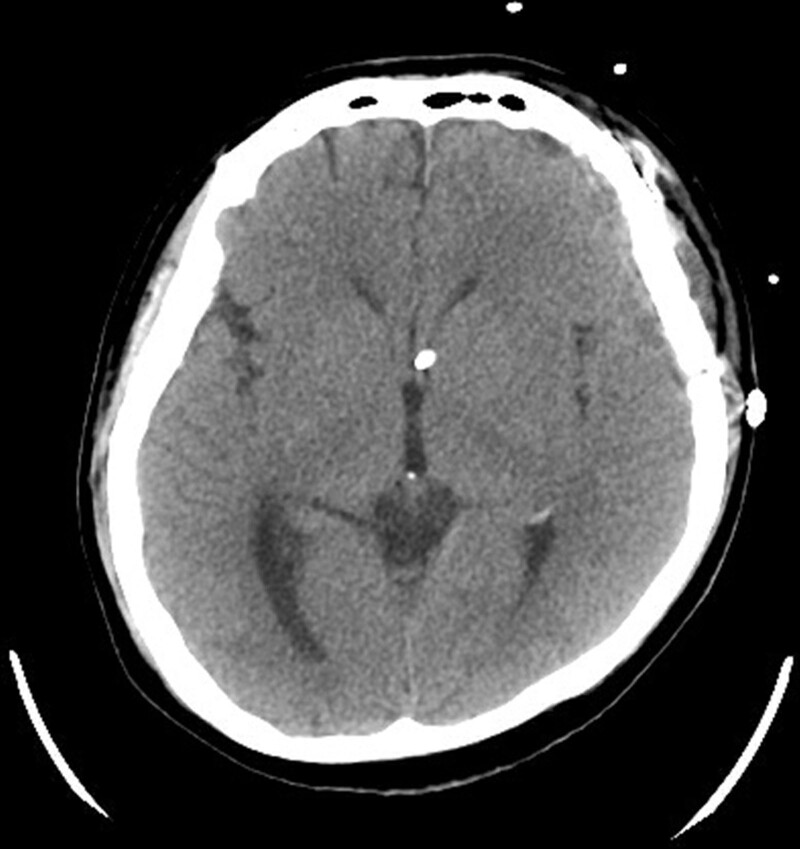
CT image on the 11th day following craniotomy. CT = computed tomography.
